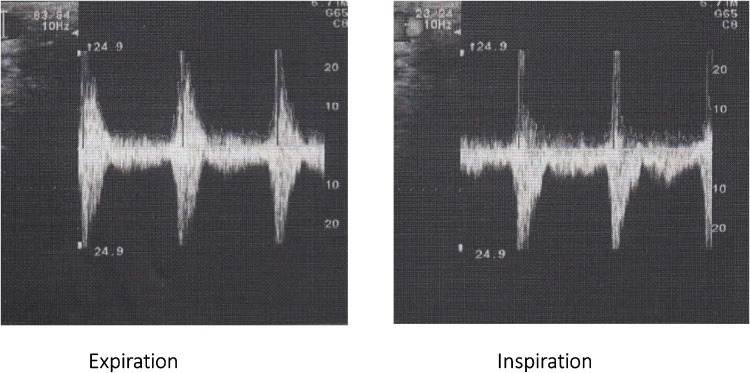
Intraoperative doppler ultrasonography showed the no difference of blood flow of celiac artery both inspiration and expiration.
